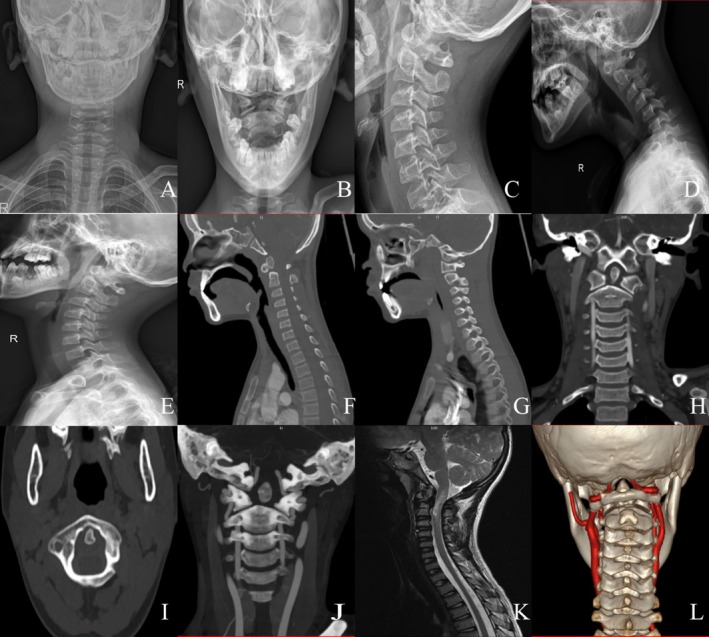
Preoperative radiographic, CT, CTA, and MRI evaluation of atlantoaxial instability/dislocation. (A) Anteroposterior cervical radiograph showing overall cervical alignment. (B) Open‐mouth odontoid radiograph showing the odontoid process and bilateral C1–C2 lateral mass joints. (C) Neutral lateral cervical radiograph showing the atlantodental interval and sagittal alignment. (D, E) Dynamic flexion–extension lateral radiographs used to evaluate atlantoaxial instability and reducibility. (F, G) Sagittal CT reconstruction showing the C1–C2 relationship and atlantoaxial alignment. (H) Coronal CT reconstruction showing the odontoid process and bilateral atlantoaxial joint alignment. (I) Axial CT image showing the odontoid process and C1 ring morphology. (J) Coronal CTA image showing the course of the vertebral arteries and their relationship with the osseous structures of the craniovertebral junction. (K) Sagittal MRI showing the spinal cord and craniovertebral junction, used to assess neural compression and associated abnormalities. (L) Three‐dimensional CTA reconstruction showing the posterior osseous anatomy and bilateral vertebral arteries. CT, computed tomography; CTA, computed tomography angiography; MRI, magnetic resonance imaging.
